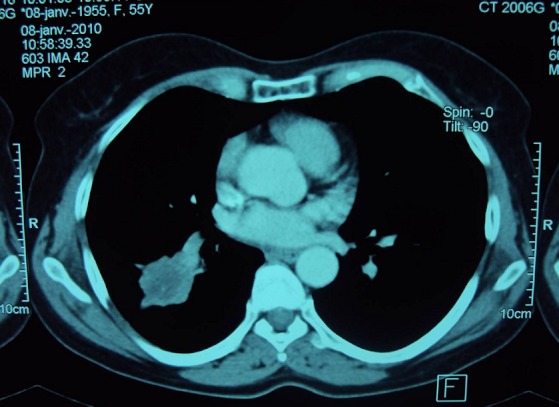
CT scan right ovalar 45x35 mm necrosed tumor with irregular margin, with no mediastinal lymph nodes, and no left parenchymatous anomaly and no pleural reaction
